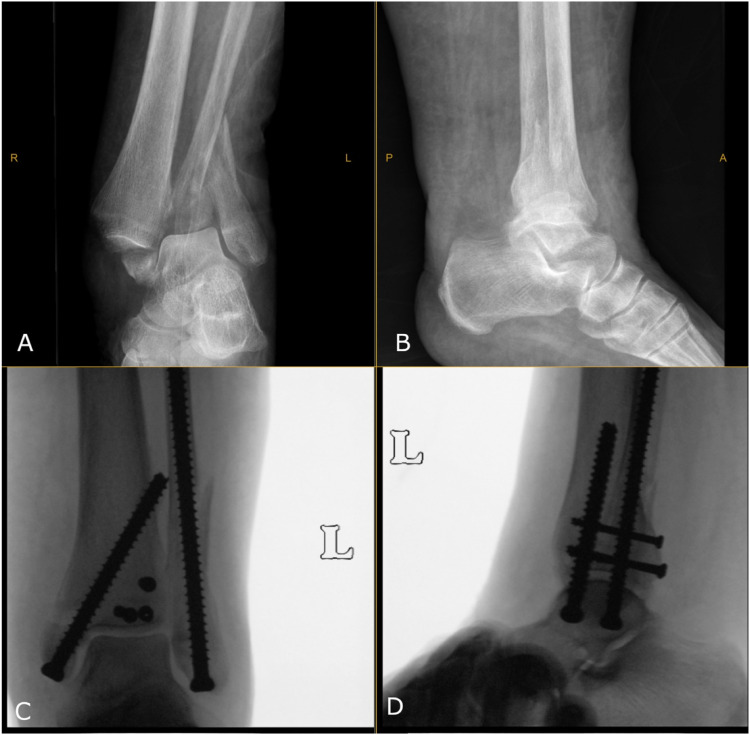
Radiographs of Case 7. Preoperative radiographs (A = anteroposterior view; B = lateral view) demonstrate a dislocated ankle joint with a trimalleolar fracture. Intraoperative image intensifier imaging (C = mortise view; D = lateral view) demonstrating excellent reduction and fixation with combination of 6.5mm and 4mm cannulated screws
